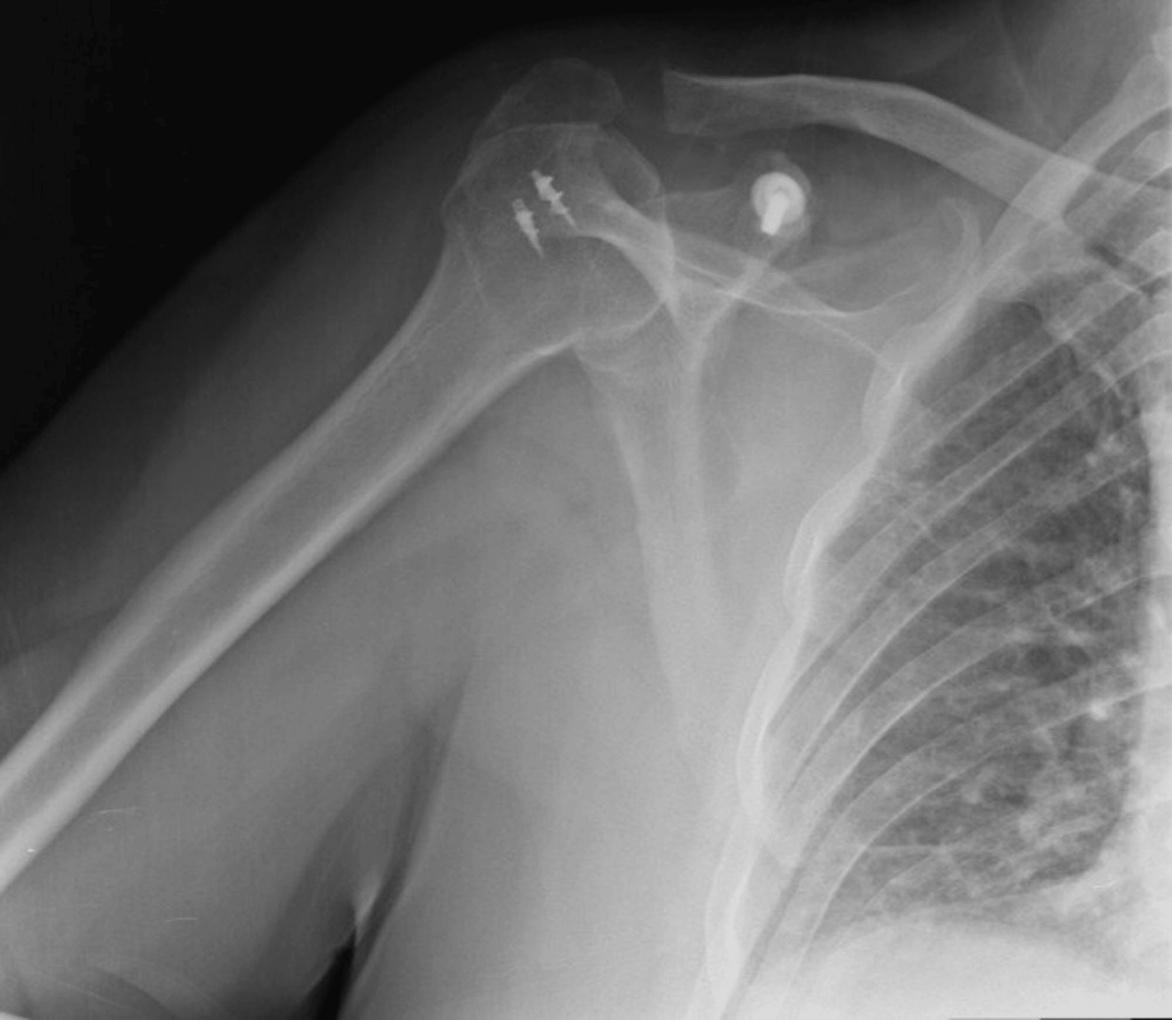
Postoperative one-month X-ray of the right shoulder joint displaying coracoid process fixation with a single screw and rotator cuff repair with two suture anchors.
